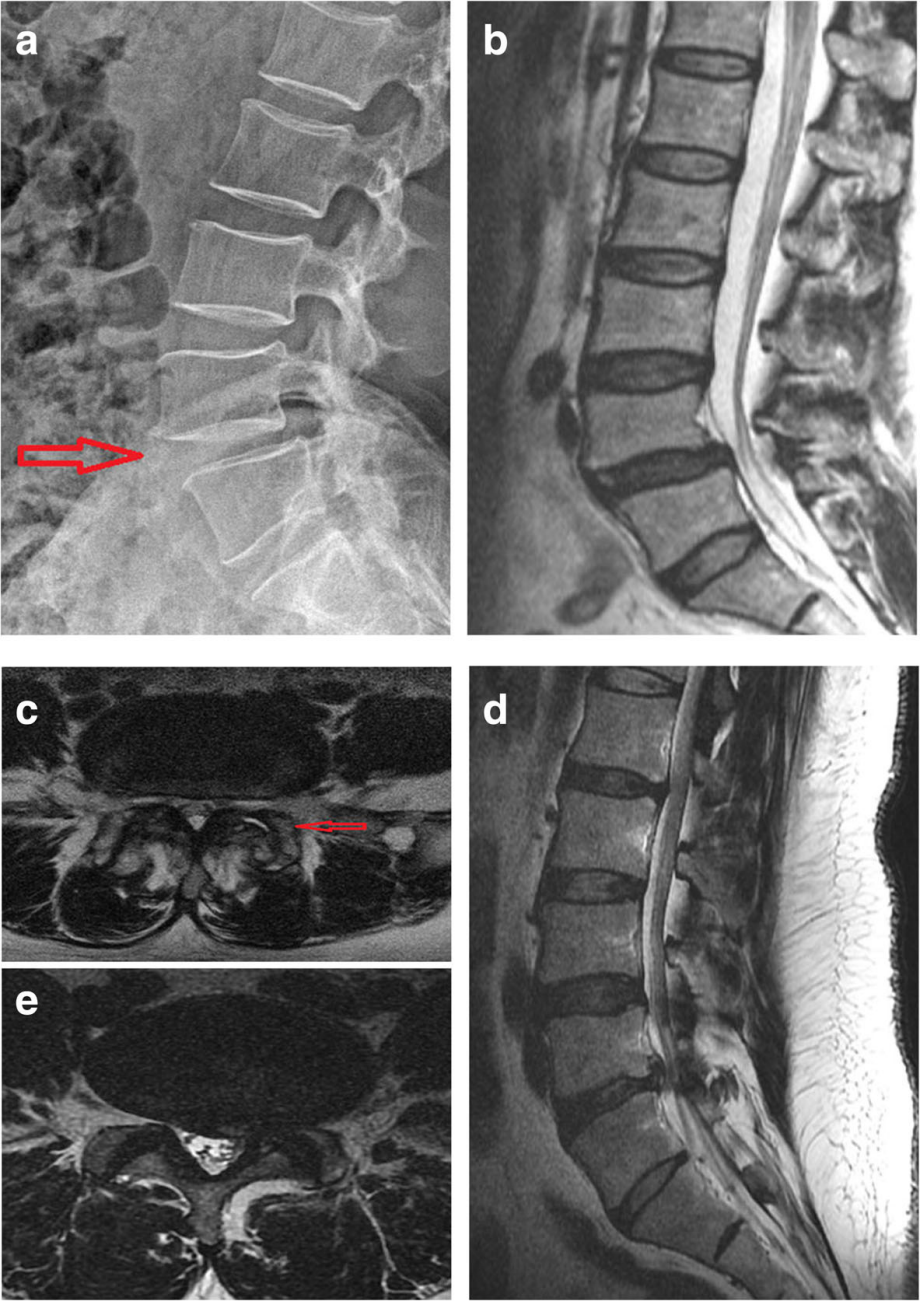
Possible causes of treatment failures in cases 3 and 6. Lumbar lateral X-ray imaging (a), T2 sagittal MRI (b), and T2 axial MRI (c) of case 3 showing degenerative spondylolisthesis of L4 on L5 (slippage or displacement of L4 vertebra compared to L5 vertebra) and spinal stenosis. T2 sagittal MRI (d) and T2 axial MRI (e) of case 9 revealed left-sided L4–5 herniated NP with decreased disc height
